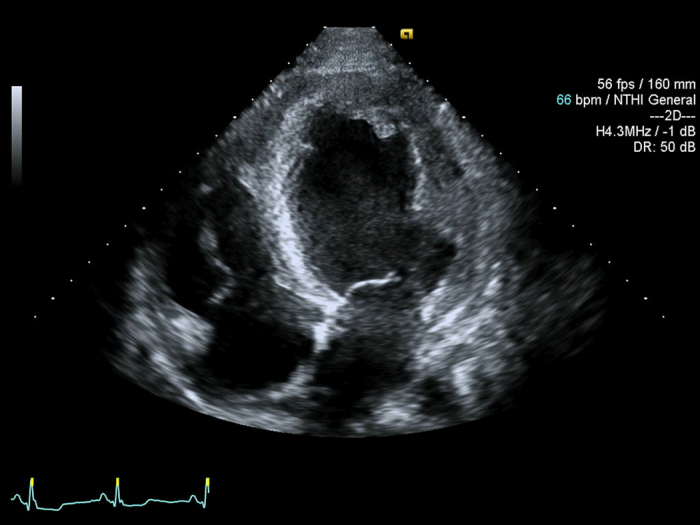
Athlete’s two dimensional echocardiographic image of left ventricle at apical 4 chamber view.
